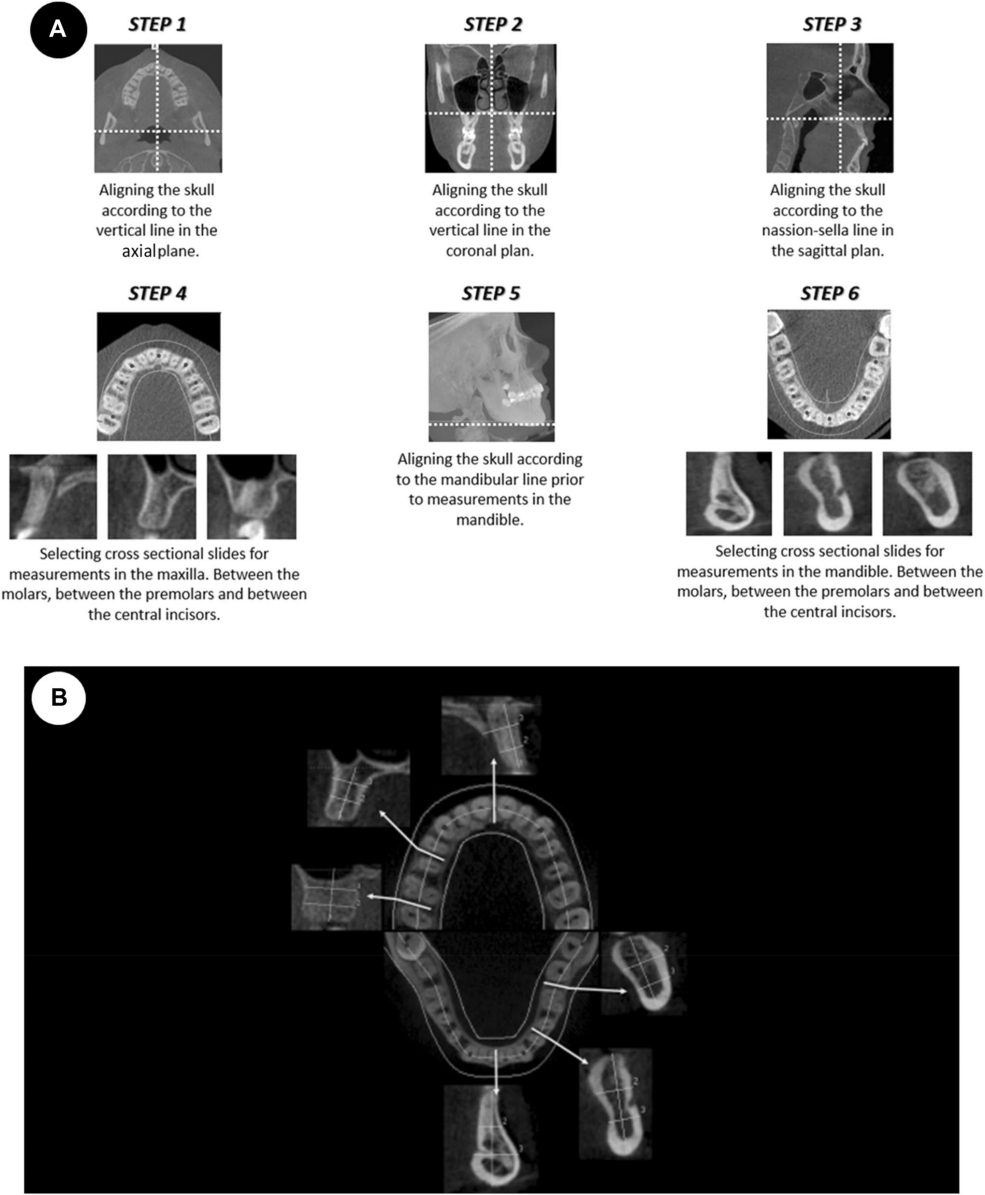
a When selecting sites for measurements, the steps performed by the raters when adjusting the volumes for small deviations in the patient´s head position during exposure. b Measurements of height and width in cross sectional CBCT images of the maxilla and mandible. One height and two width measurements at each site were measured. The sites were named according to location of the neighboring teeth, e.g. upper molar = UM
